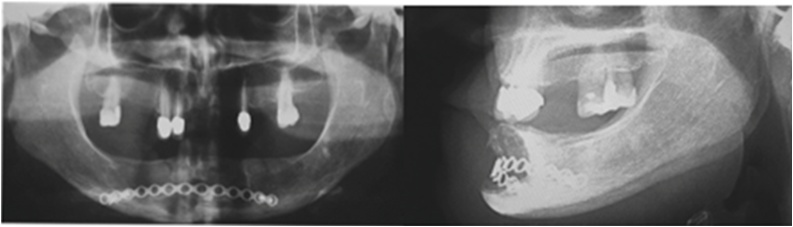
Orthopantomography 3 months after surgical procedure and it was visualized an establish process of healing.
